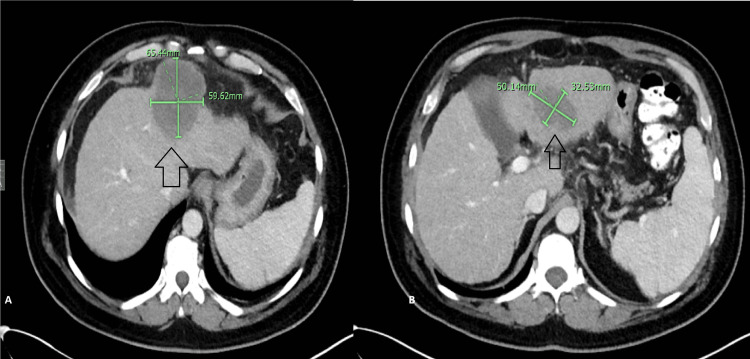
March 2020 CT scan of the abdomen Radiological evidence of response to 5-FU, oxaliplatin, and pembrolizumab with a relative reduction in the size of both left hepatic lobe masses is noted (arrows in A and B) 5-FU: 5-Fluorouracil
